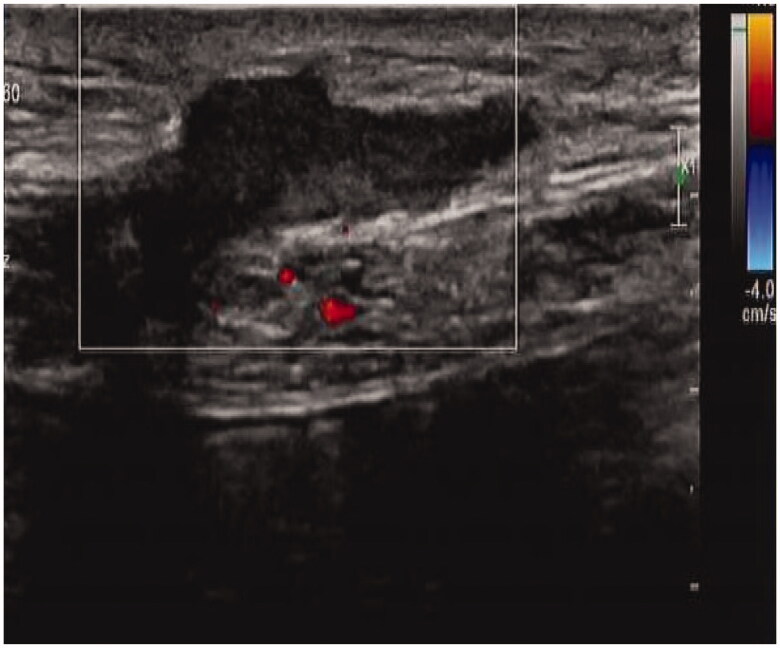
B-Mode Ultrasound Image of case 1.
